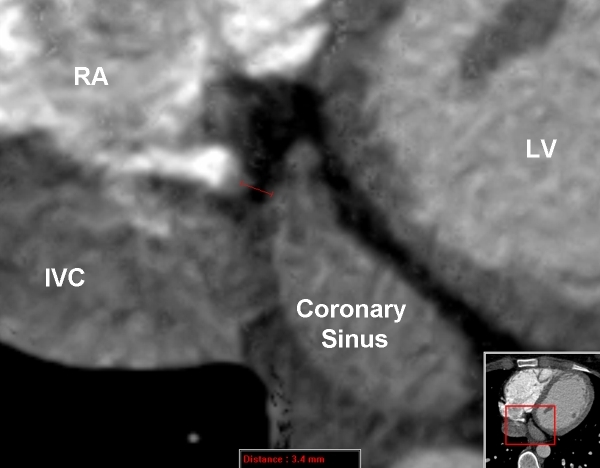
Magnified CT scan image showing coronary sinus ostial atresia. The CS is seen to taper abruptly and end approximate 3.4 mm before reaching the right atrium (see figure 3 for comparison). Abbreviations: RA = right atrium, LV = left ventricle, IVC = inferior vena cava
